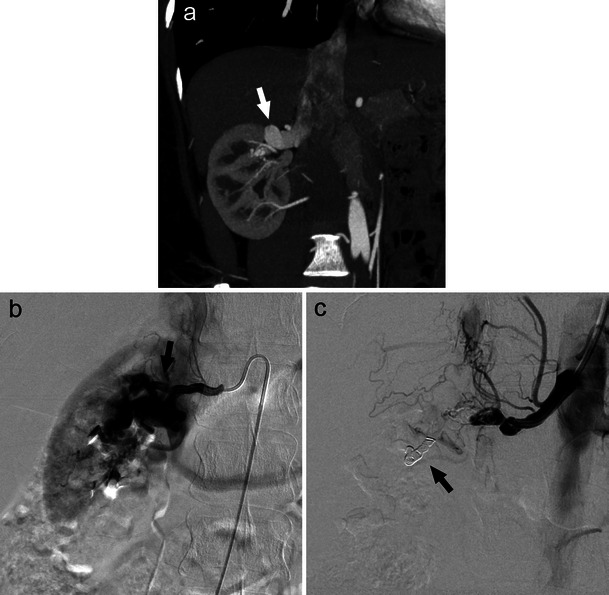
Right RAVM as seen on CT (a, white arrow) and angiogram (b, black arrow). Coil embolisation provides an effective option in the treatment of larger RAVMS (c, black arrow)
